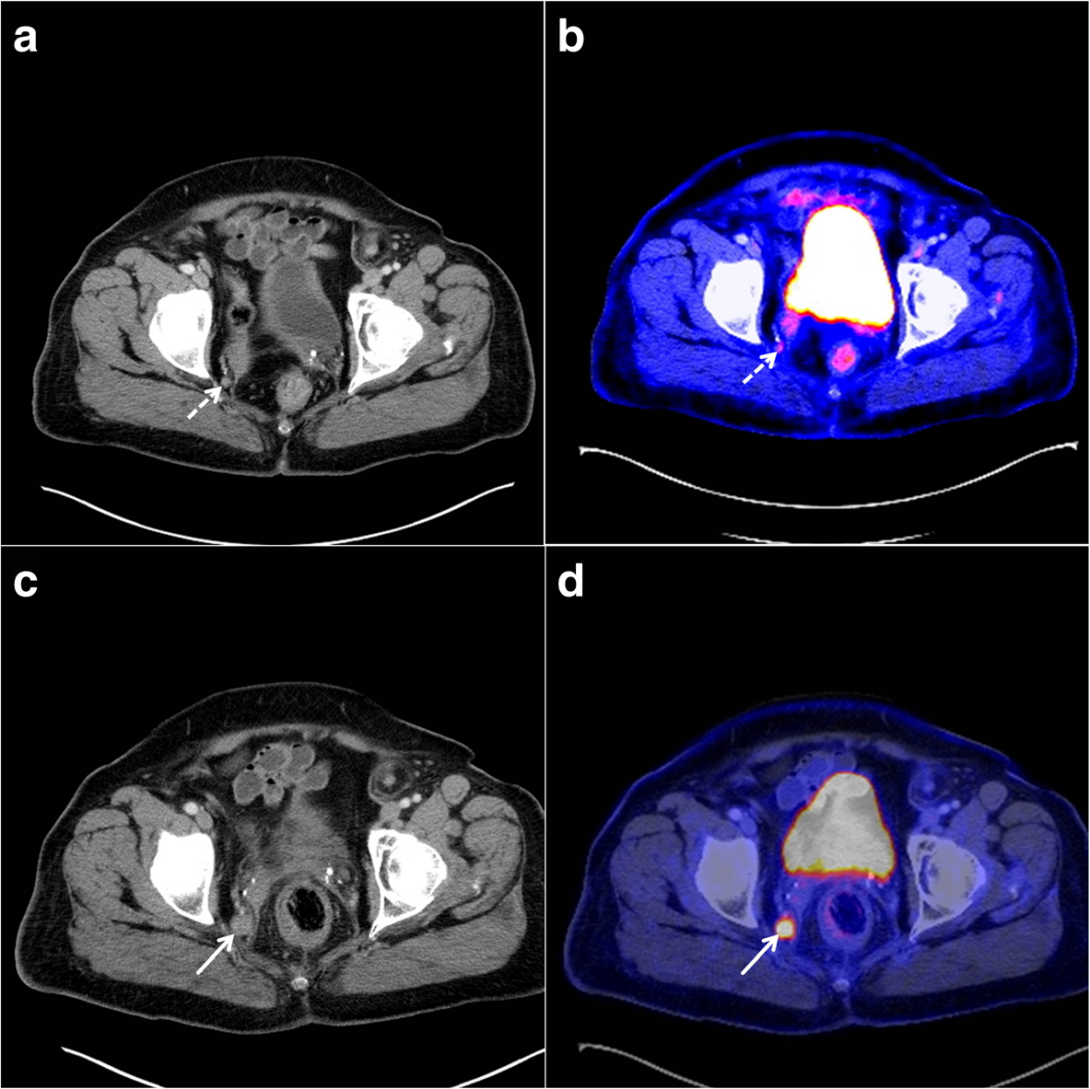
CT and PET/CT for pelvic imaging in anorectal malignant melanoma. A seventy-three-year-old female patient (*patient 5*) with a small iliacal lymph node metastasis on the right side (*dashed arrow*) and a relapse from anal melanoma. Contrast-enhanced CT reveals a small lymph node beside the right internal iliac artery of round configuration with uncertain dignity (a). PET/CT, however, indicates increased FDG uptake suspicious for metastasis (b). The follow up examination 9 months later provides clear evidence of progressive lymphatic metastasis on the right iliac side (*arrow*) on CT (c) and PET/CT (d) images. The lymph node is enlarged with a destroyed anatomical structure and increased glucose uptake. PET/CT enabled detection of lymph node metastasis in an early state
